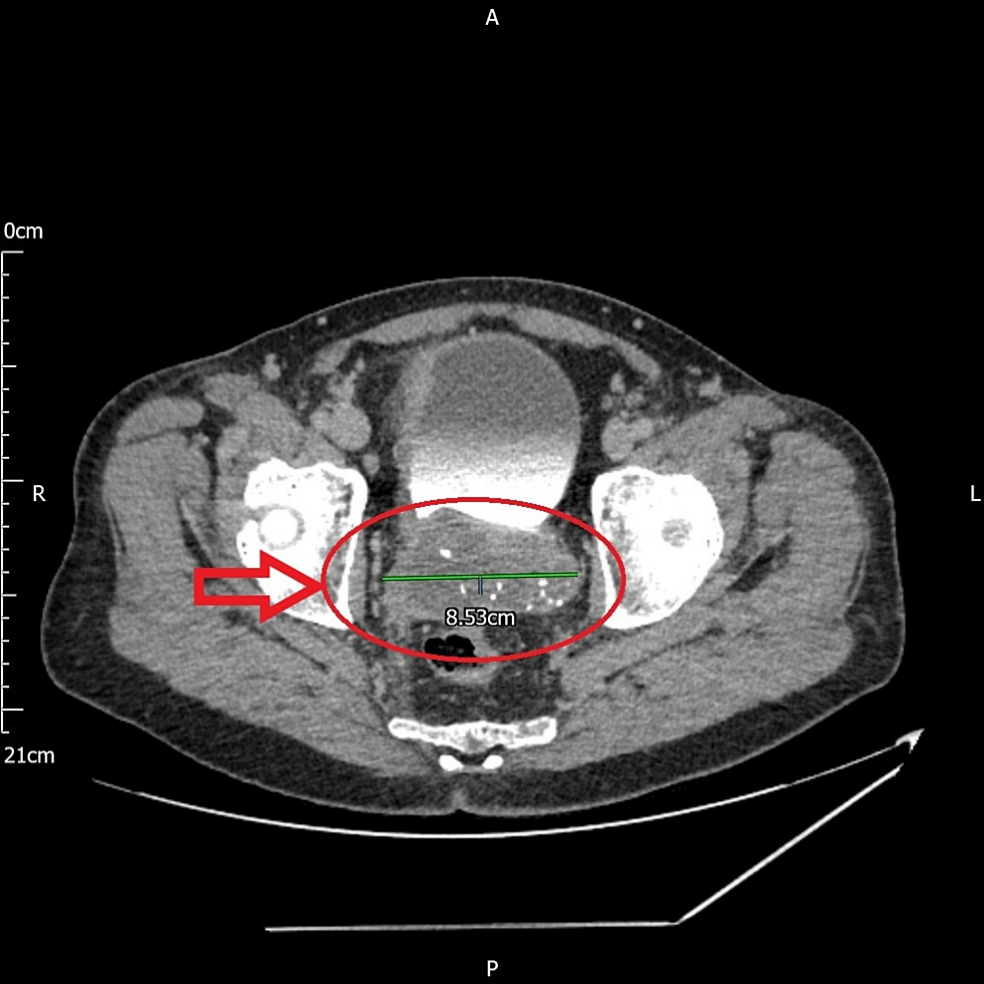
CT urogram showing enlarged seminal vesicles having mixed density with cystic components, heterogeneous enhancement, and coarse calcification
